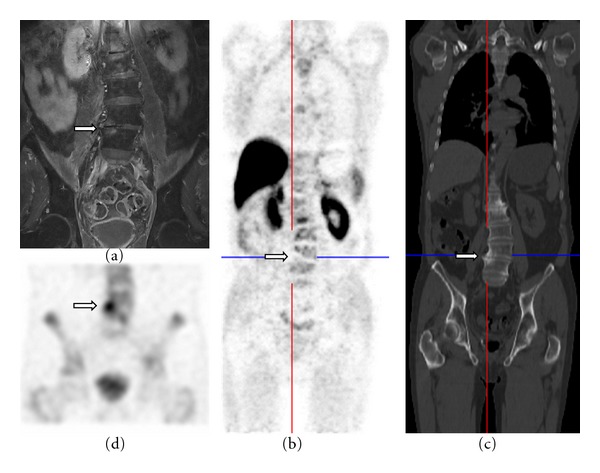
(a) MRI T2-weighted coronal plane shows a degenerative change in the lumbar spine at the level of L4/5. (b), (c) ^18^F-FECH-PET/CT (coronal planes). (d) Bone scan (SPECT).
